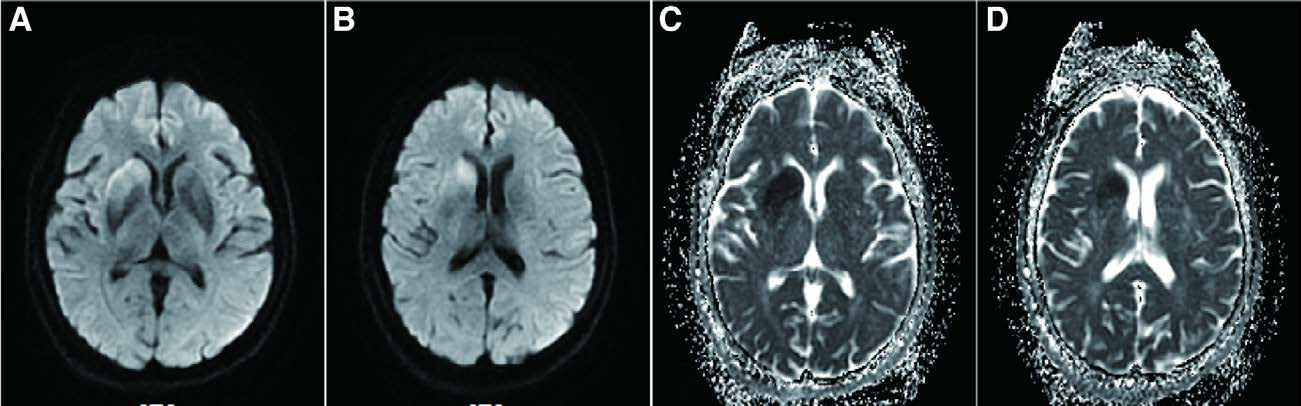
Diffusion weighted imaging (DWI) revealed diffusion restriction at the right putamen and caudate nucleus (A and B) and apparent diffusion coefficient (ADC) at the corresponding position (C and D).
